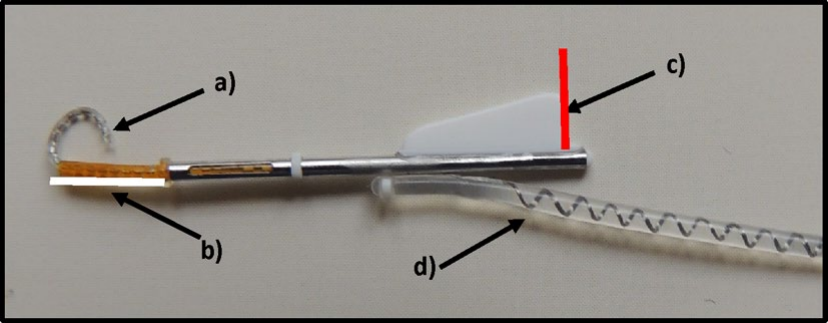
Cochlear™ Nucleus^®^ CI 532 Slim Modiolar practice cochlear implant. The major parts of the device: a Cochlear implant electrode, b Insertion Guide (IG; white line), c Orientation marker (OM; red line), d Electrode lead. These lines (white and red) are marked on the subsequent CT scan (Fig. 2a) and 3D model (Fig. 3a and b) for easier orientation
